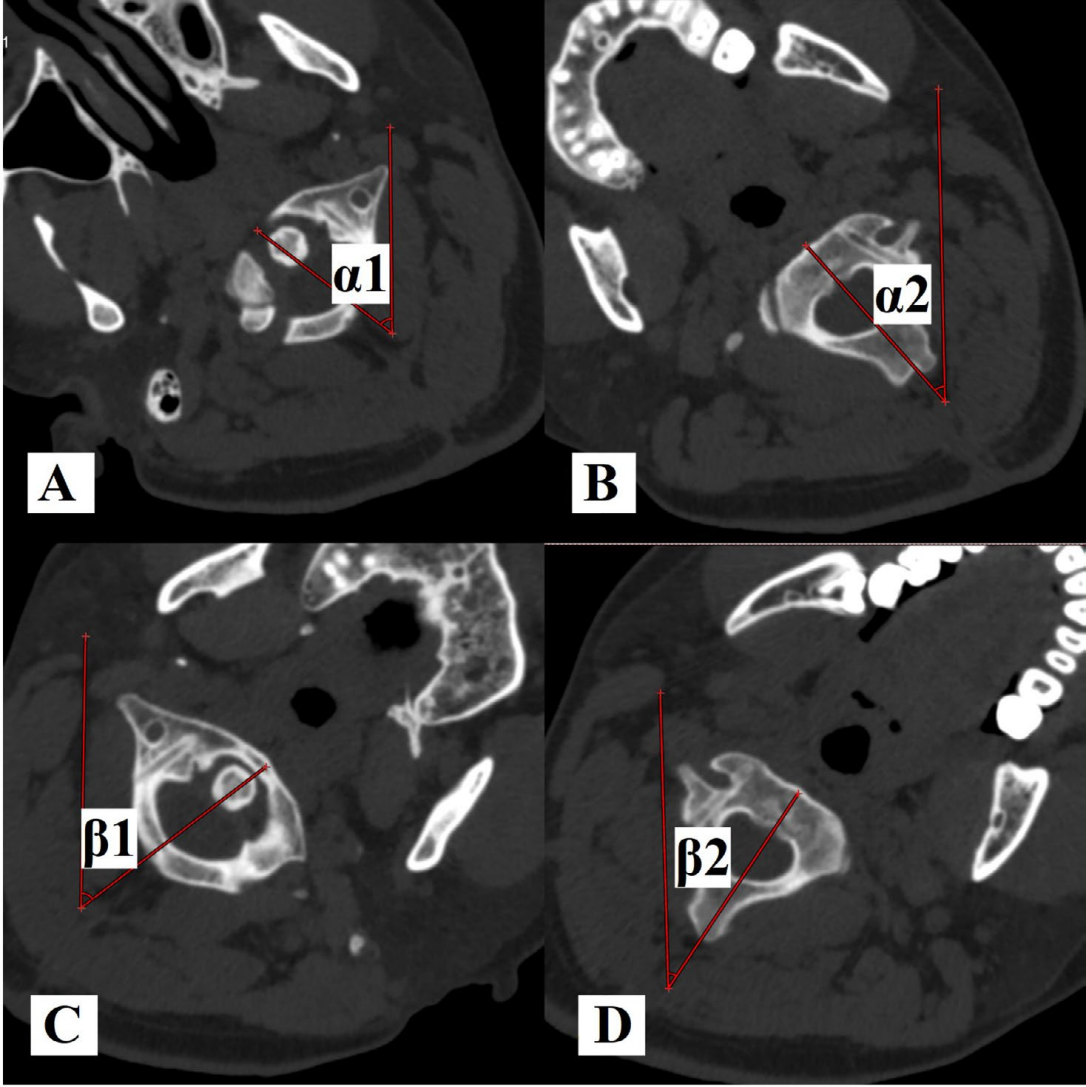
Measurements of ROM in rotation of C1–C2 on rotational CT scan, left side, α1–α2 = 15°, right side, β1–β2 = 20°.
